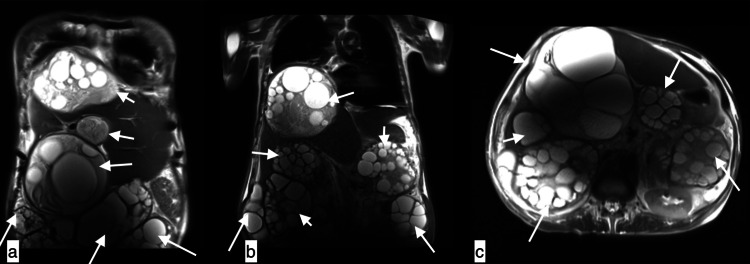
Peritoneal hydatidosis A 52-year-old male with peritoneal hydatidosis presented with progressively increasing abdominal distension, weight loss, and anorexia. Coronal (a,b) and axial (c) MRI abdomen showing multiple cystic lesions with peripherally arranged daughter cysts of varying sizes in the right lobe of the liver, subhepatic space, and visualized peritoneal cavity causing superior displacement of the diaphragm and causing compression of the right lower lobe. Cysts in the peritoneal cavity are compressing the pancreatic head and tail, bilateral kidneys with right minimal pleural effusion.
